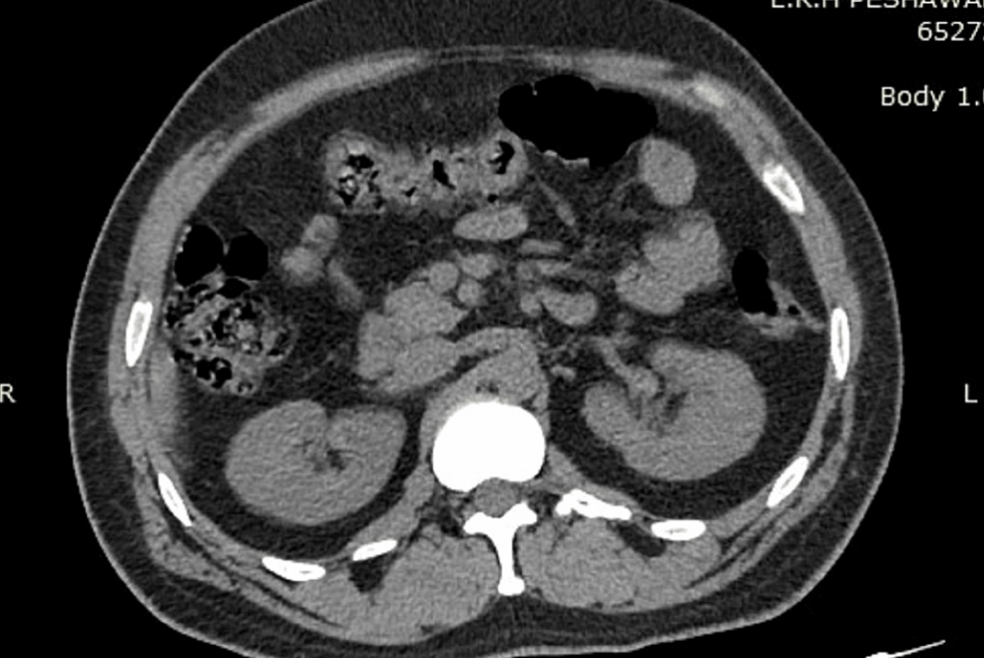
Postoperative CT KUB image (stone clearance achieved using Swiss LithoClast Trilogy). CT KUB = computed tomography of the kidneys, ureters, and bladder
